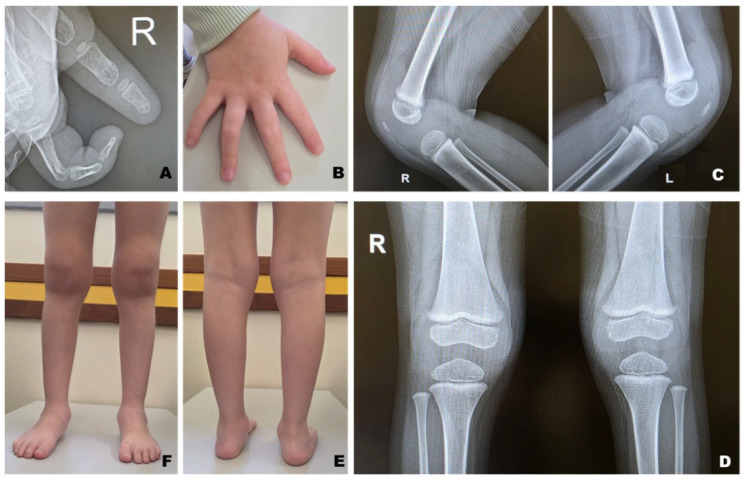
Clinical and radiological features of the patient with camptodactyly–arthropathy–coxa vara–pericarditis (CACP) syndrome. (A) Radiograph of the right hand showing residual camptodactyly of the index finger, with persistent flexion at the proximal interphalangeal joint and preserved bone structure, without erosions. (B) Clinical photograph of the hands demonstrating mild camptodactyly, more evident in the third finger of the right hand, associated with slender, tapered fingers, with no signs of local inflammation. (C) Lateral radiographs of both knees showing periarticular soft tissue enlargement consistent with joint effusion, without erosive changes. (D) Anteroposterior radiographs of both knees demonstrating metaphyseal widening of the distal femora and proximal tibiae, producing a characteristic Erlenmeyer flask–like deformity. (E) Posterior view of the lower limbs showing involvement of the knees, ankles, and feet, with flat feet and symmetrical hindfoot alignment. (F) Anterior view of the lower limbs demonstrating symmetrical, painless swelling of the knees and ankles, without external inflammatory signs.
